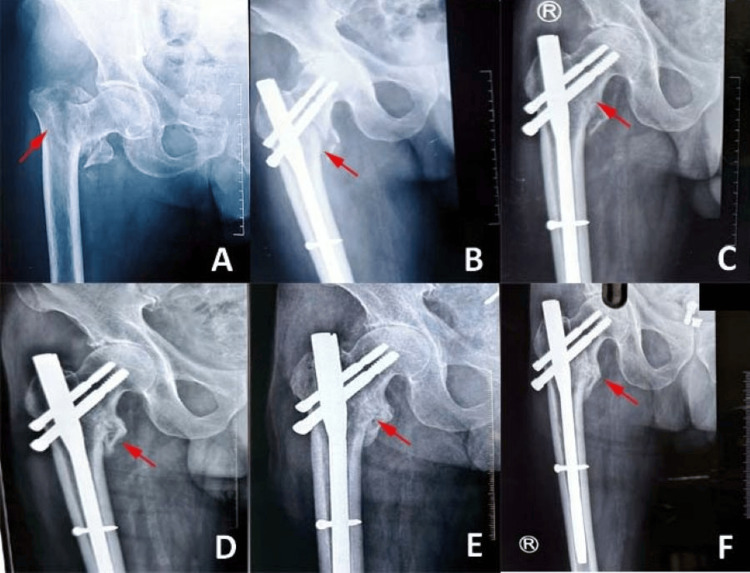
Serial radiographs demonstrating postoperative implant position and fracture healing: (A) Preoperative radiograph showing intertrochanteric fracture; (B) Immediate postoperative (day 0) radiograph demonstrating implant placement; (C) Early follow-up (day 14) showing maintained alignment; (D) Progressive healing (week 6th) with stable fixation; (E) Advanced healing (month 3) with callus formation; and (F) Late follow-up (9th month) showing complete healing and stable implant. Original radiographic images of the patient from the study, used with the patient's permission.
